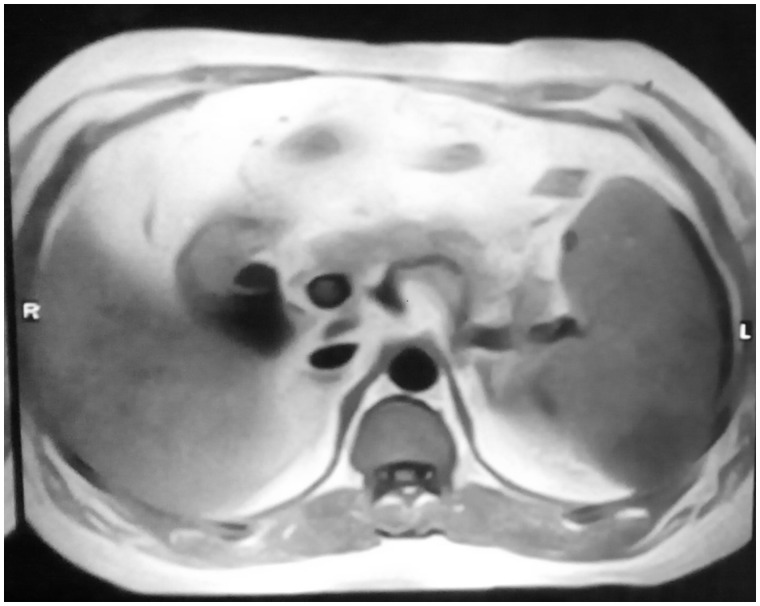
Magnetic resonance cholangiopancreatography showing a hyperintense area within the spleen on T2-weighted imaging, with splenomegaly
